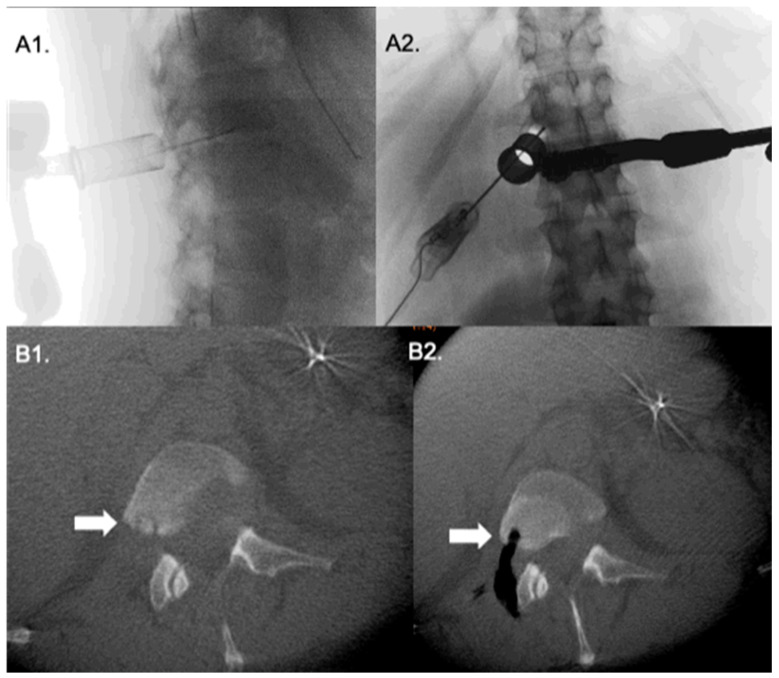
Transforaminal approach to to a tumor in the posterior aspect of L1 vertebral body. Lateral and antero-posterior fluoroscopy (A1,A2) show the tubular retractor with a probe pointing to the lesion site. Intraoperative O-arm CT axial cuts (B1,B2) before and after tumor resection.
